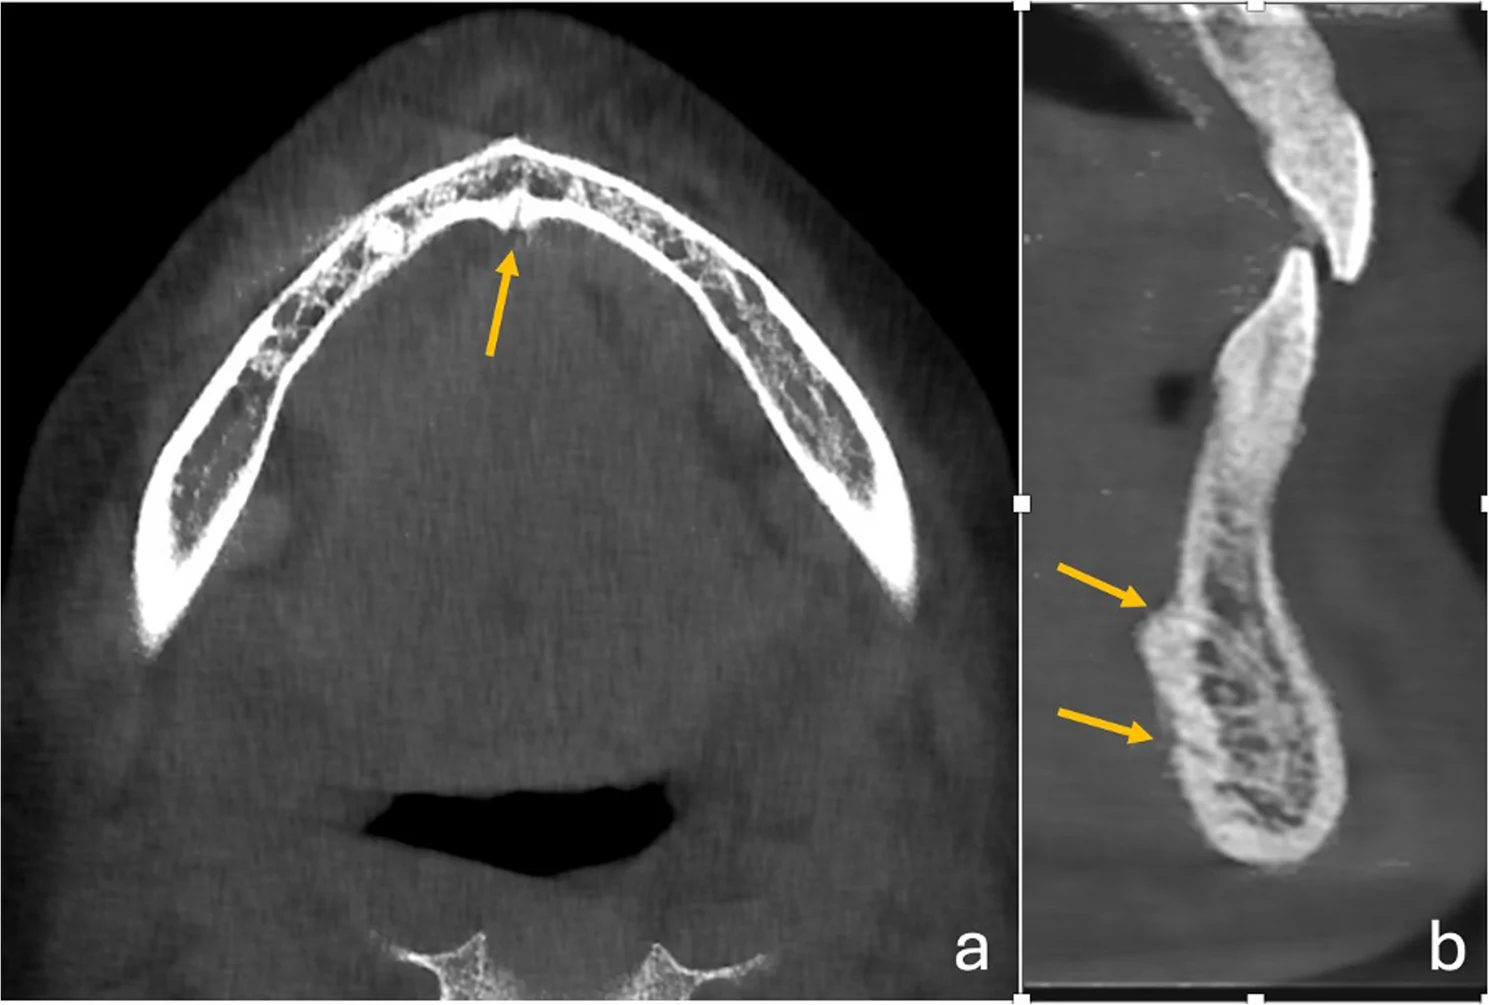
Multi-planar identification and counting of mandibular lingual foramina. a Axial CBCT view showing the entry point of the lingual foramen on the mandibular midline. b Corresponding cross-sectional (sagittal) view of the same patient, where arrows demonstrate the vertical arrangement of multiple foramina, allowing for an accurate count and independent measurement of each canal
